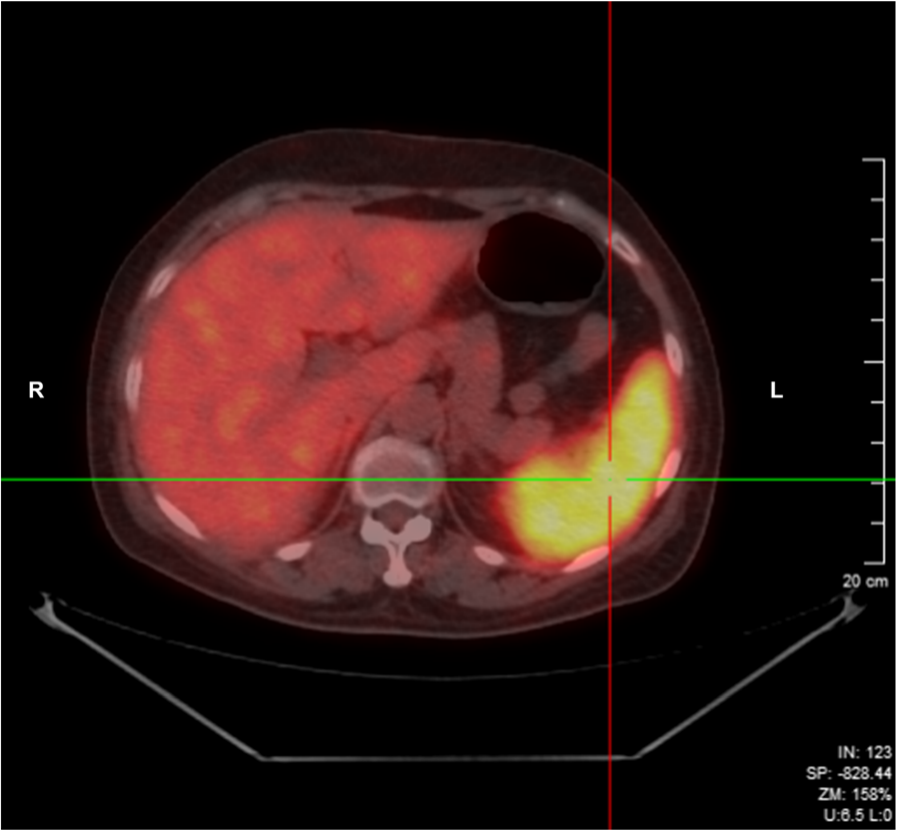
PET-CT of spleen. PET-CT scan revealed spleen abnormality with SUV_max_ 10.2
